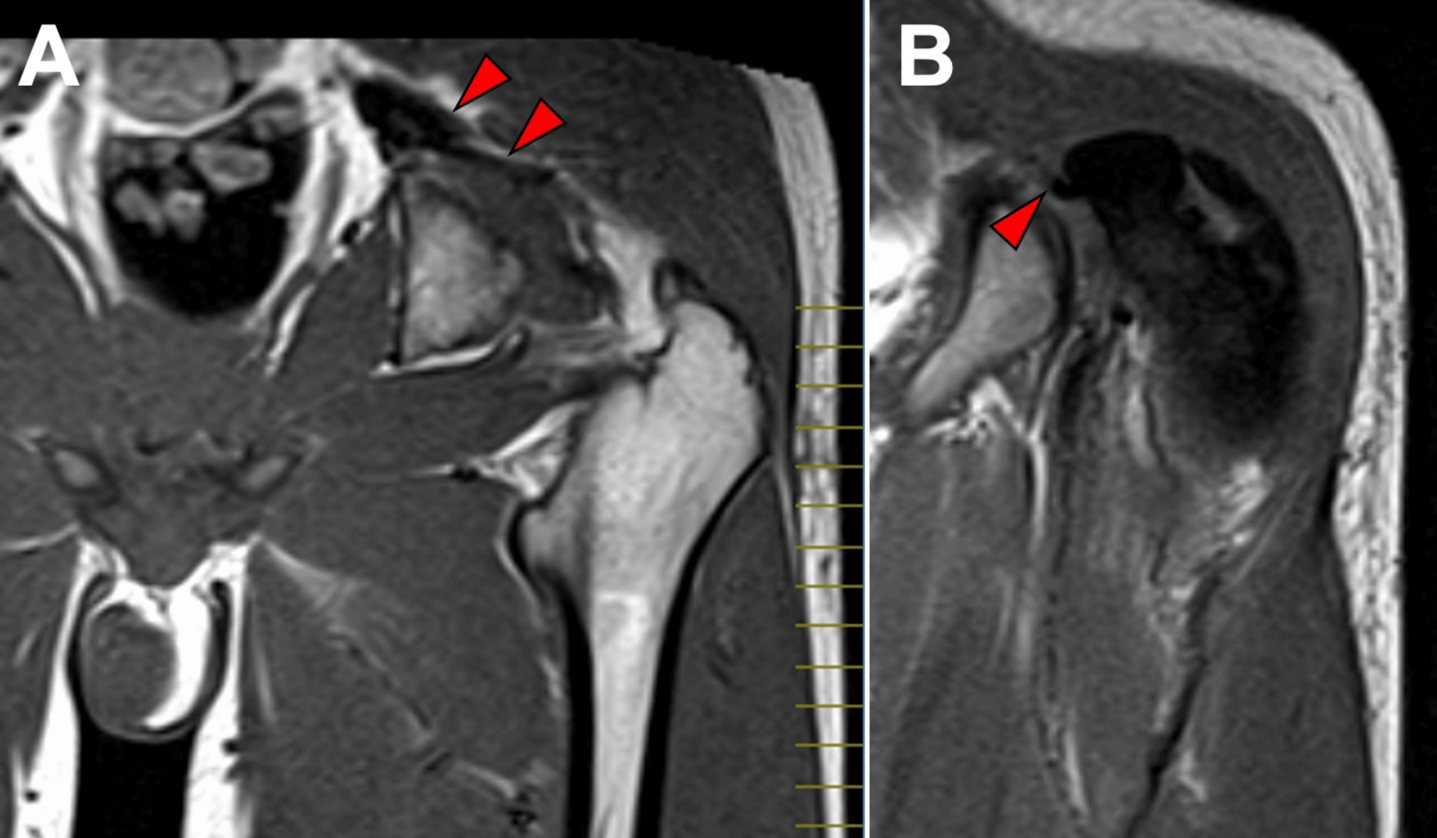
Initial MRI at the referring hospital: coronal T1-weighted images. Dilation of the left internal iliac artery extending to the inferior gluteal artery (panel A, red arrows) and a contiguous intramuscular signal-void area (panel B, red arrow) within the left gluteus maximus is observed.
